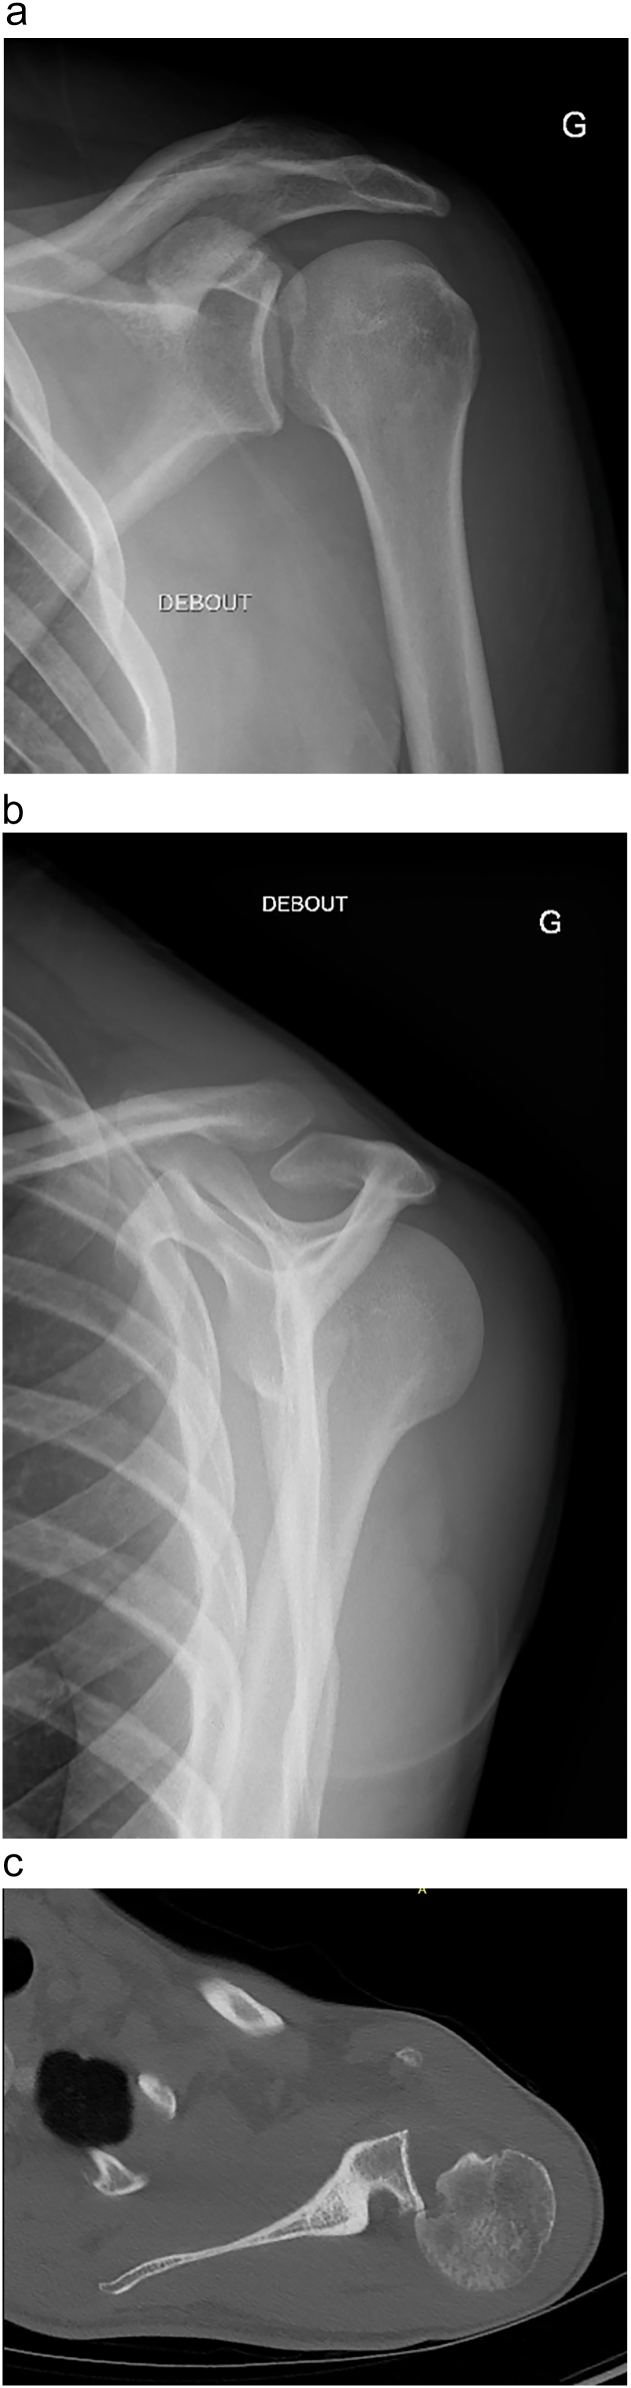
a, b: X-rays showing left shoulder posterior dislocation. c: CT scan showing Posterior left shoulder dislocation with reverse hill Sachs lesion.
